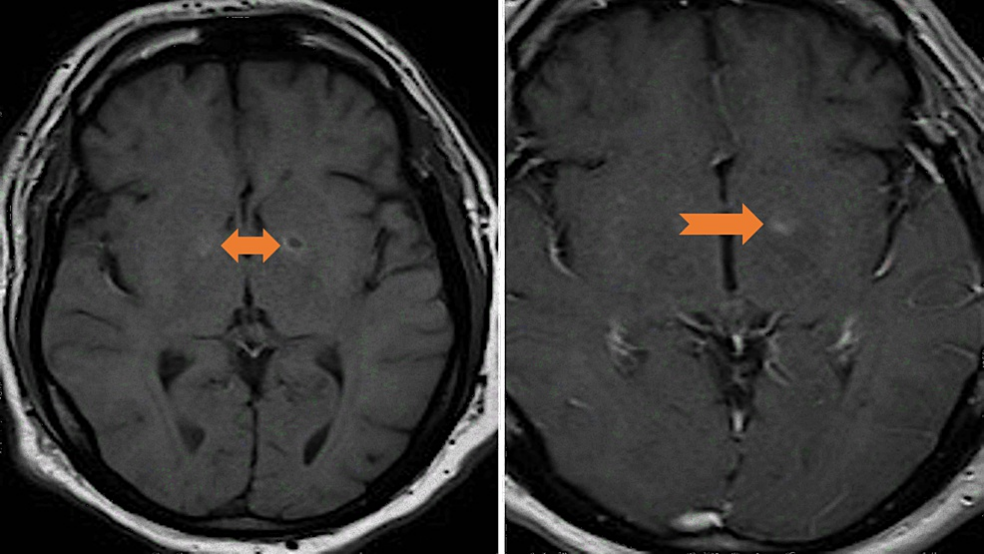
MRI of the brain T1 sequence axial images with and without contrast. Mild restricted diffusion and patchy non-mass-like enhancement in portions of this signal abnormality without mass effect (arrows). MRI: magnetic resonance imaging
